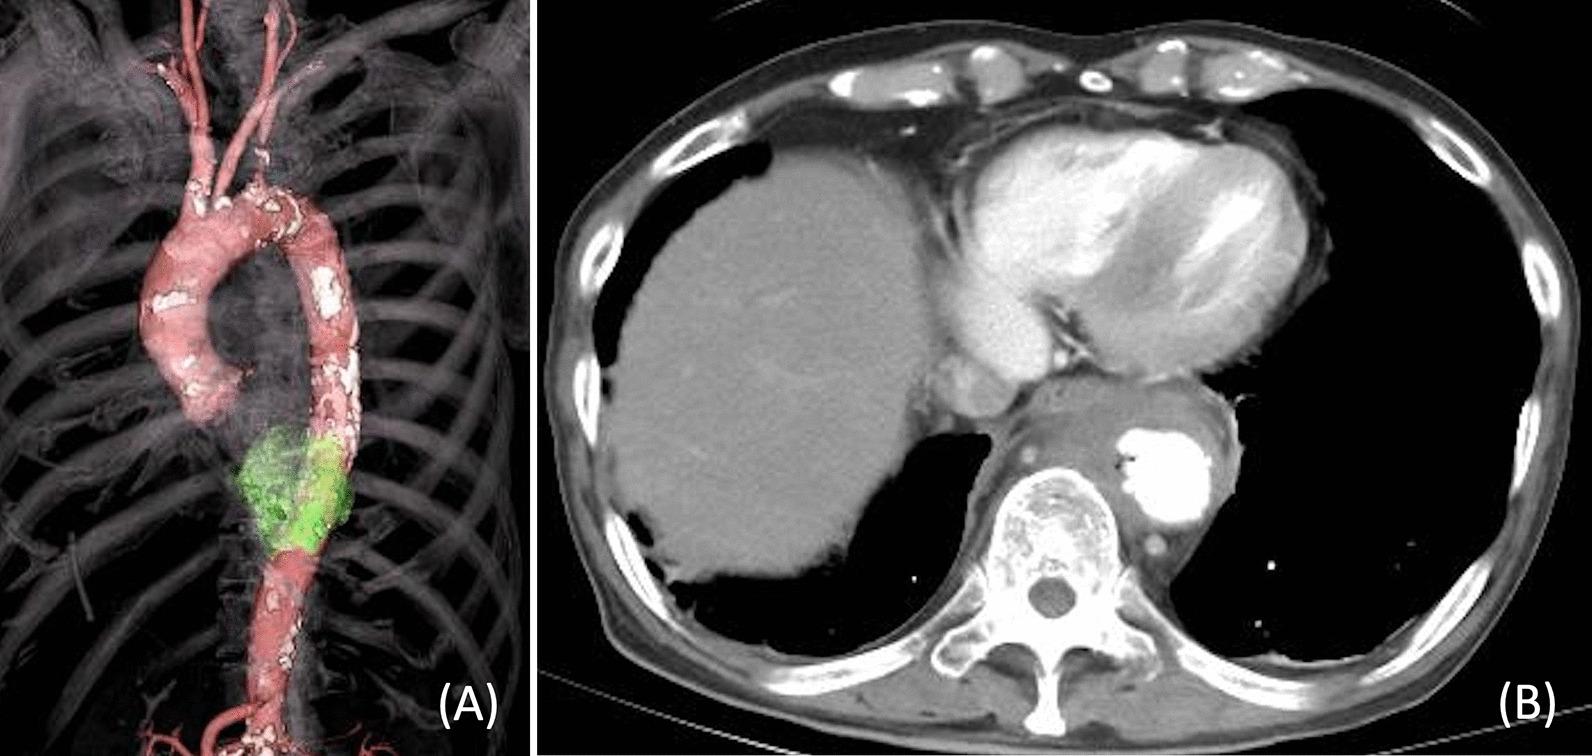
Computed tomography (CT) before the first thoracic endovascular aortic repair (TEVAR) reveals a ruptured pseudoaneurysm in the descending thoracic aorta (at the level of Th10) surrounded by fluid accumulation (A: 3D CT; B: contrast-enhanced CT)
